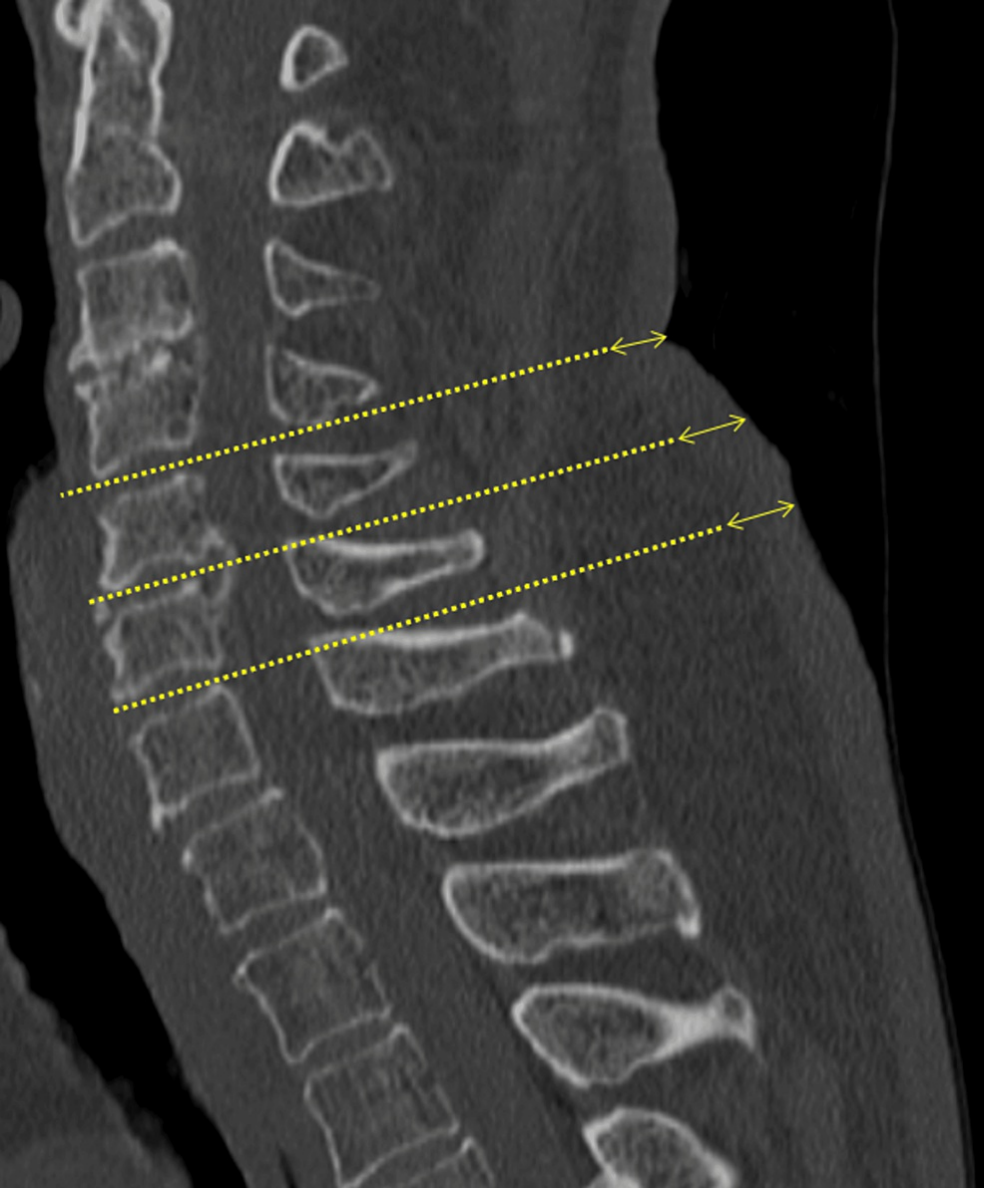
Measurement of cervical spine skin thickness using sagittal whole-spine CT. Dashed line: extension of the intervertebral disc. Bidirectional arrows: skin thickness. In this case, the skin thickness (average) of the cervical skin is 7.7 mm (7.3 mm at the C4-5 level, 8 mm at the C5-6 level, and 7.9 mm at the C6-7 level).
